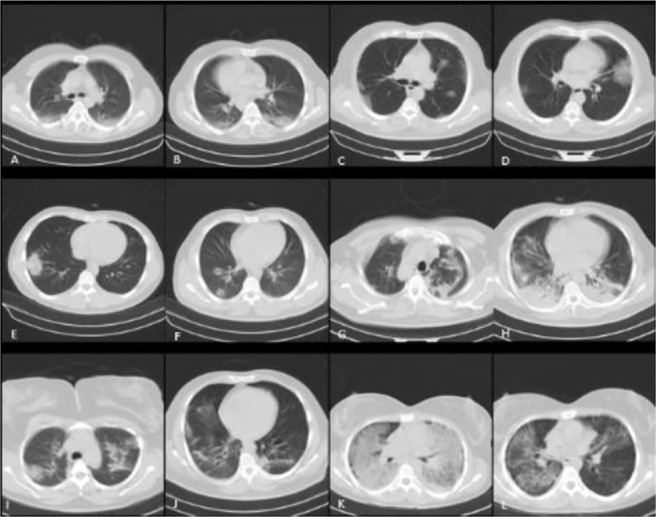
Various appearances of SARS-Cov-2 in CT scan of patients with positive PCR tests and respiratory symptoms: A–B: Typical peripherally located GGO, C–D: GGO in both peri-bronchovascular and peripheral locations. E: isolated consolidation without air-bronchogram sign. F: nodular appearance of GGO in subpleural part of right lower lobe. G: irregular bands affecting apex with consolidation suggestive for sub-acute phase of infection. H: Bi-basilar consolidations resembling aspiration pneumonia. I–J: consolidations with an irregular border and sub-pleural bands. K: diffuse GGO with air-bronchogram and bilateral pleural effusion, this pattern is atypical for SARS-Cov-2. L: GGO with halo sign; Subpleural sparing is also evident as subpleural lucency.
